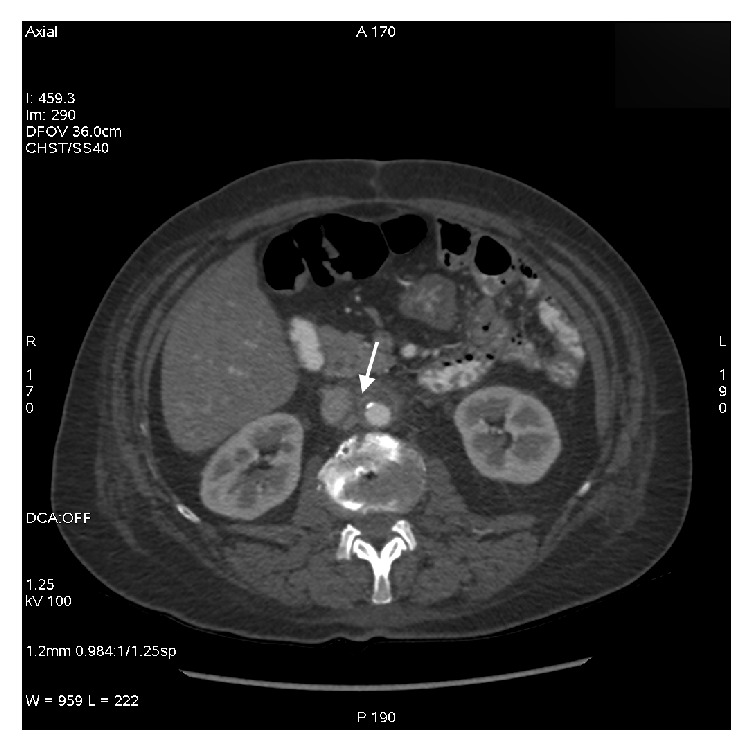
Abdominal CT scan (day 1) revealing infrarenal periaortic infiltrates in contact with the inferior vena cava* (arrow)*.
